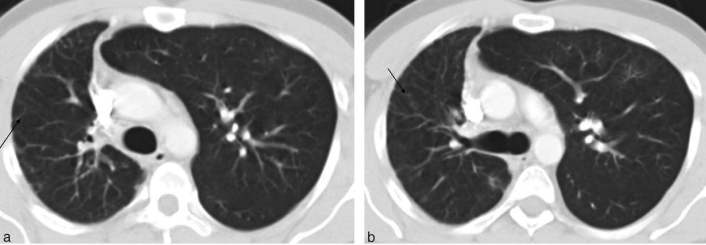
CT scans in lung window setting. The fine reticular opacities within the small right lung represent transpleural collateral vessels [
